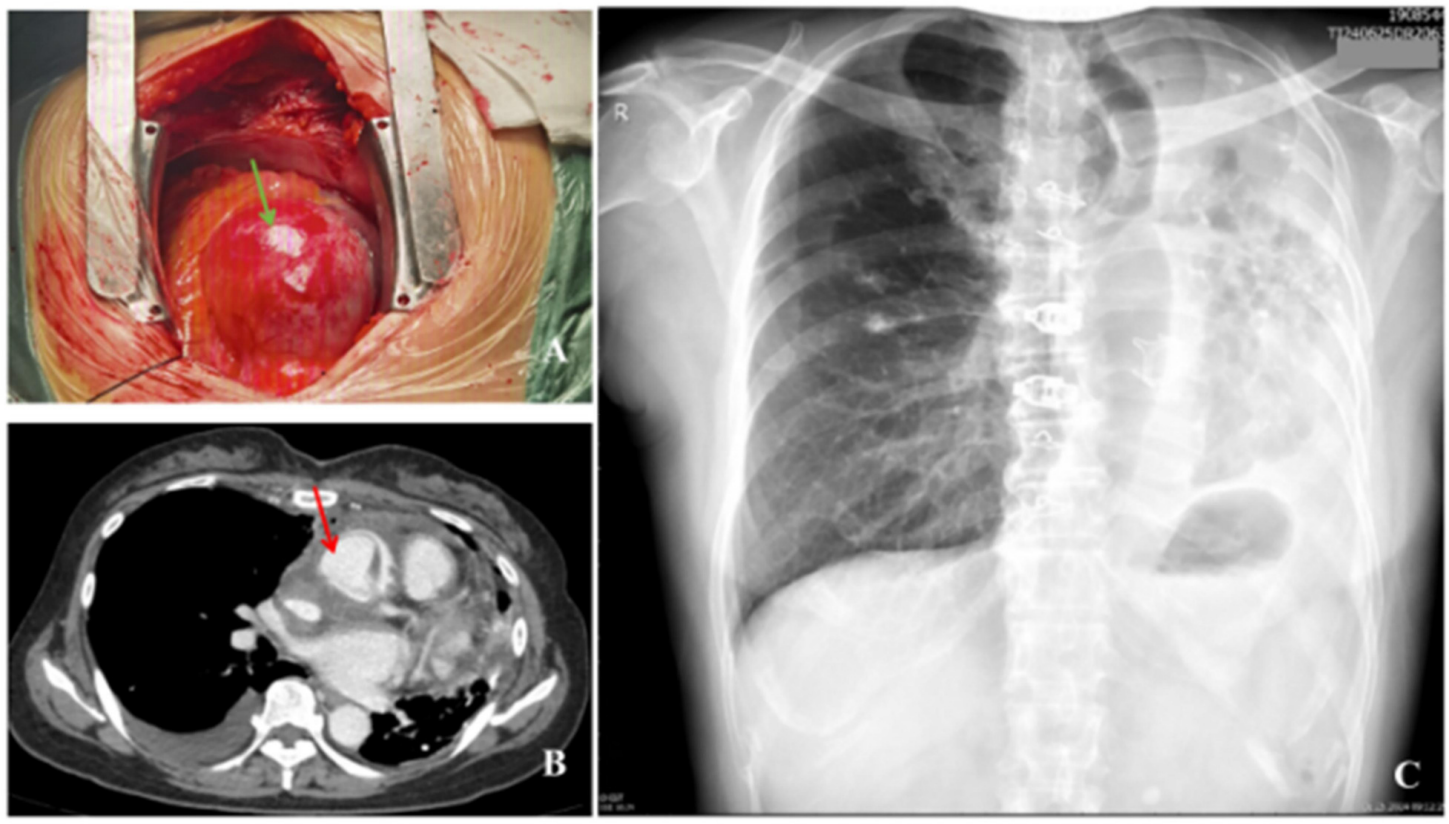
Intraoperative findings and postoperative cardiovascular CTA and chest x-ray images. (A) The aneurysm observed during the operation (indicated by the green arrow). (B) Postoperative changes of the ascending aorta graft seen on chest CTA (indicated by the red arrow), with no significant changes in the left lung destruction. (C) Chest X-ray 1 month after surgery shows left lung destruction, compensatory emphysema in the right lung, and postoperative changes.
